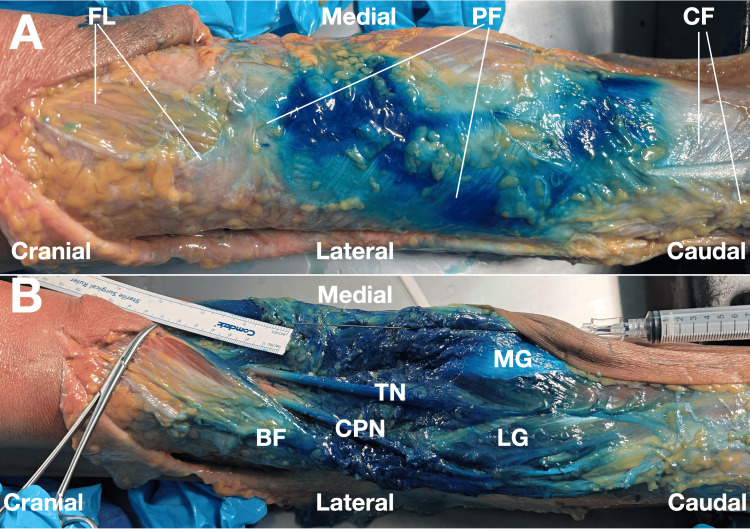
Gross dissection photographs of methylene blue distribution following ultrasound-guided injection targeting the popliteal fascial retinaculum. (A) After removal of the skin and subcutaneous adipose tissue, gross methylene blue distribution to the popliteal fascia is shown before incision of the deeper fascial layers. Dye is visible within the superficial aspects of the popliteal fascial retinaculum.
